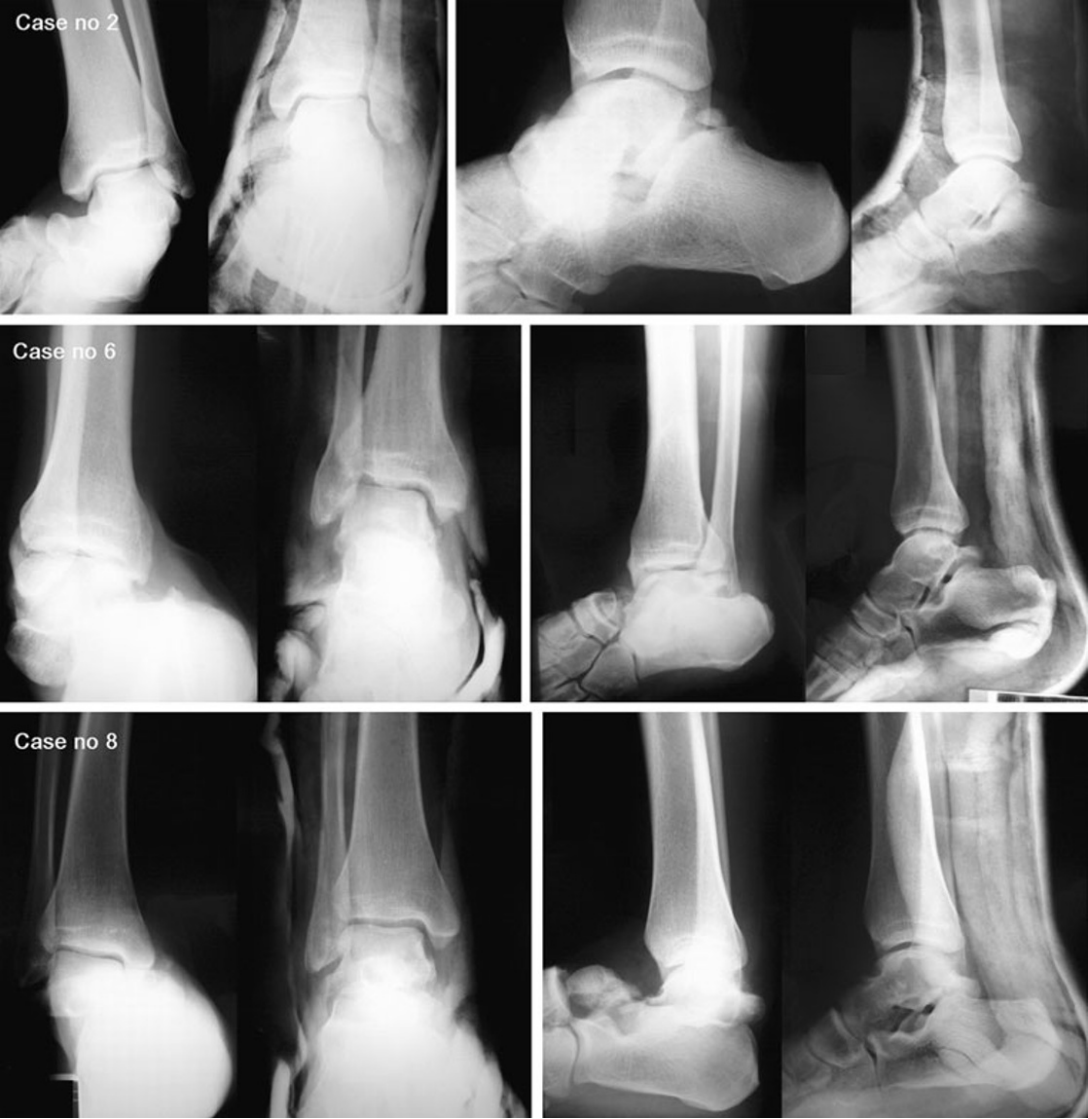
Pre- and postreduction anteroposterior and lateral radiographs of cases 2, 6, and 8
